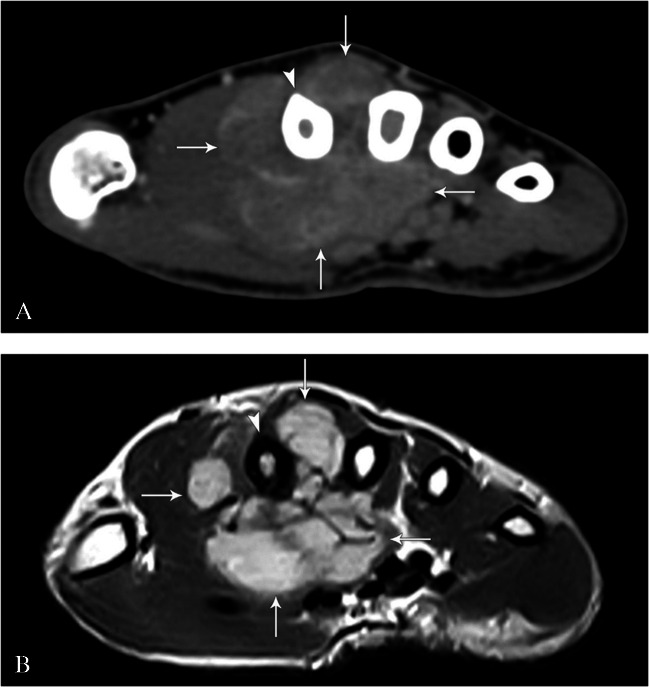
29-year-old male with Ewing sarcoma of the second metacarpal. Axial contrast-enhanced CT (A) and axial T2 (B) MRI images demonstrate a large extraskeletal mass (arrows) without any identifiable cortical disruption (arrowheads), also known as the wraparound sign
